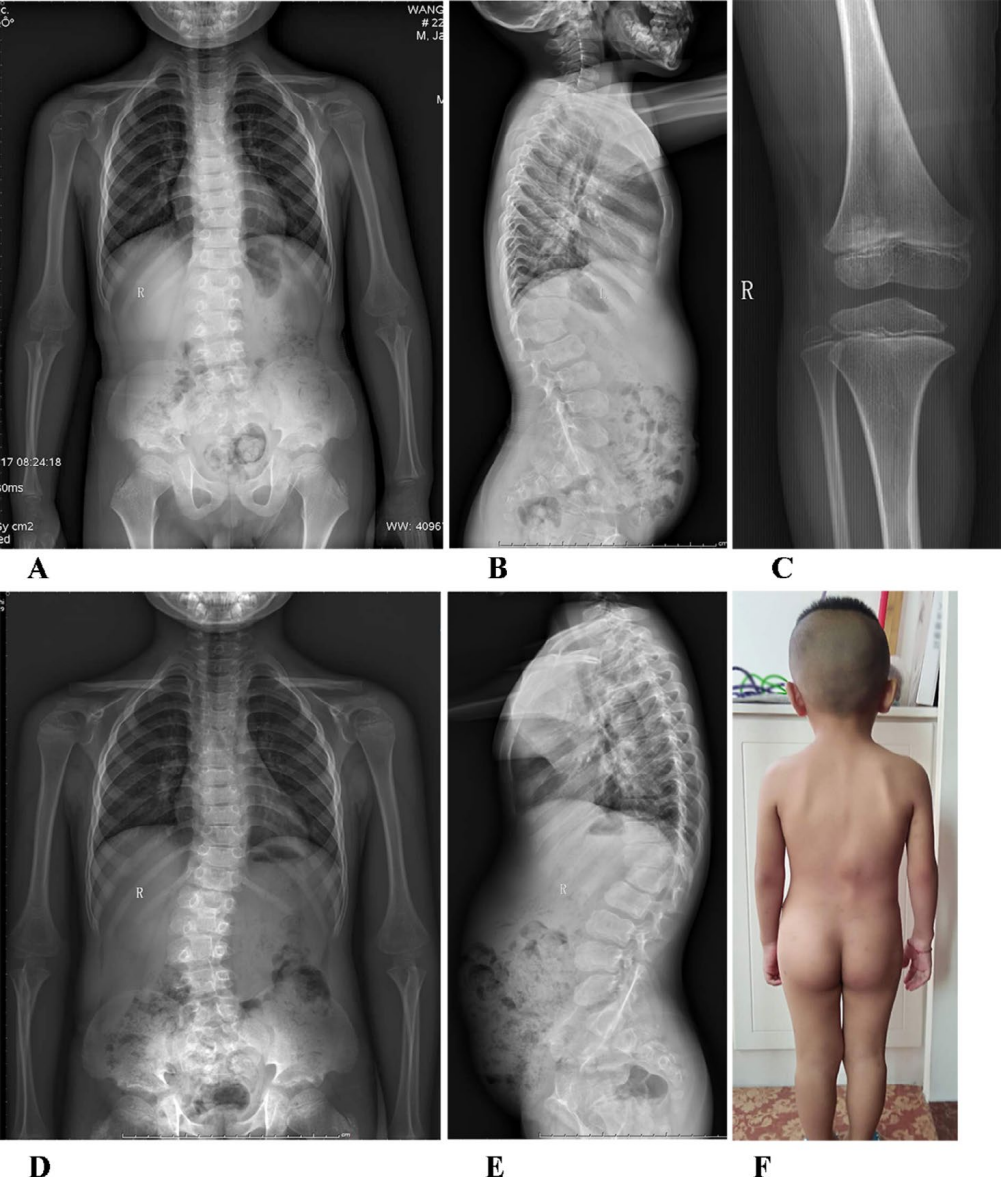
Clinical and radiographic manifestations of the child before growth hormone treatment at the age of 5 years and 6 months and stopping growth hormone treatment at the age of 7 years 9 months. (A, B) Anterior and lateral views of the spine at the age of 5 years and 6 months. (C) Anterior view of the knee at the age of 5 years and 6 months. (D, E) Anterior and lateral views of the spine at the age of 7 years and 9 months. (F) Clinical graph of the child at the age of 7 years and 9 months.
